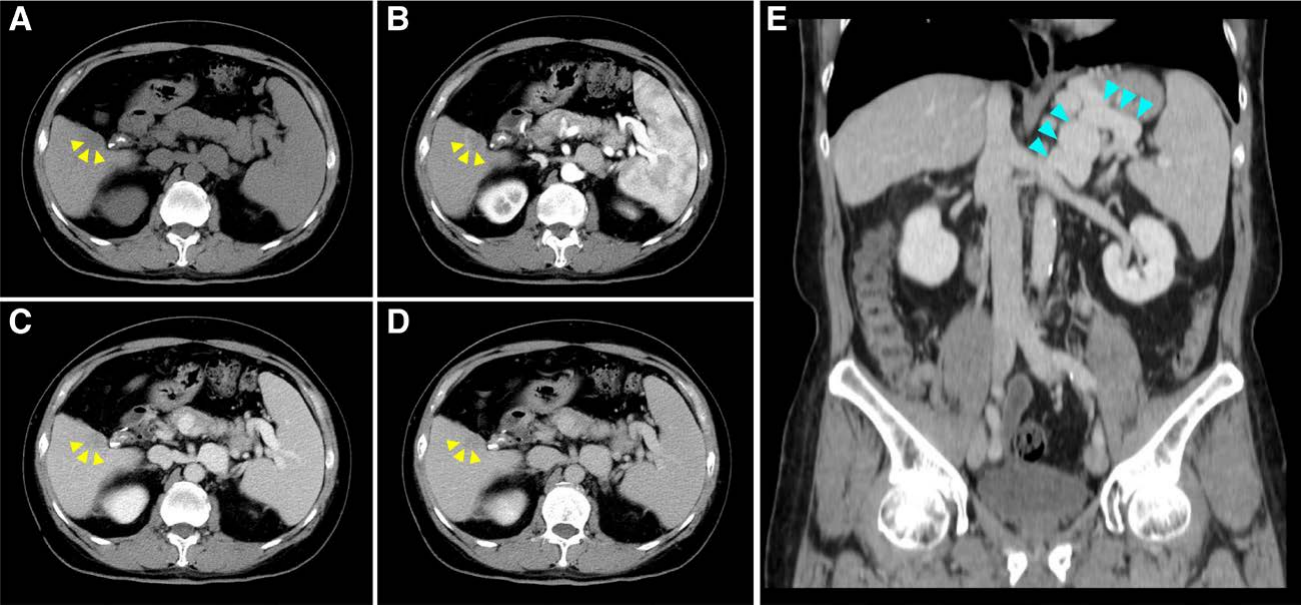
Triple-phase abdomen and pelvis computed tomography before partial hepatectomy. (A) Pre-contrast. (B) Arterial phase. (C) Portal phase. (D) Delayed phase of computed tomography showed an early enhance and early washout nodule (yellow arrowhead) in segment 5 of the liver, compatible with hepatocellular carcinoma. (E) Splenomegaly measured by size of 13.95 cm and a huge splenorenal shunt and varices (blue arrowhead) were clearly demonstrated in coronal plane.
